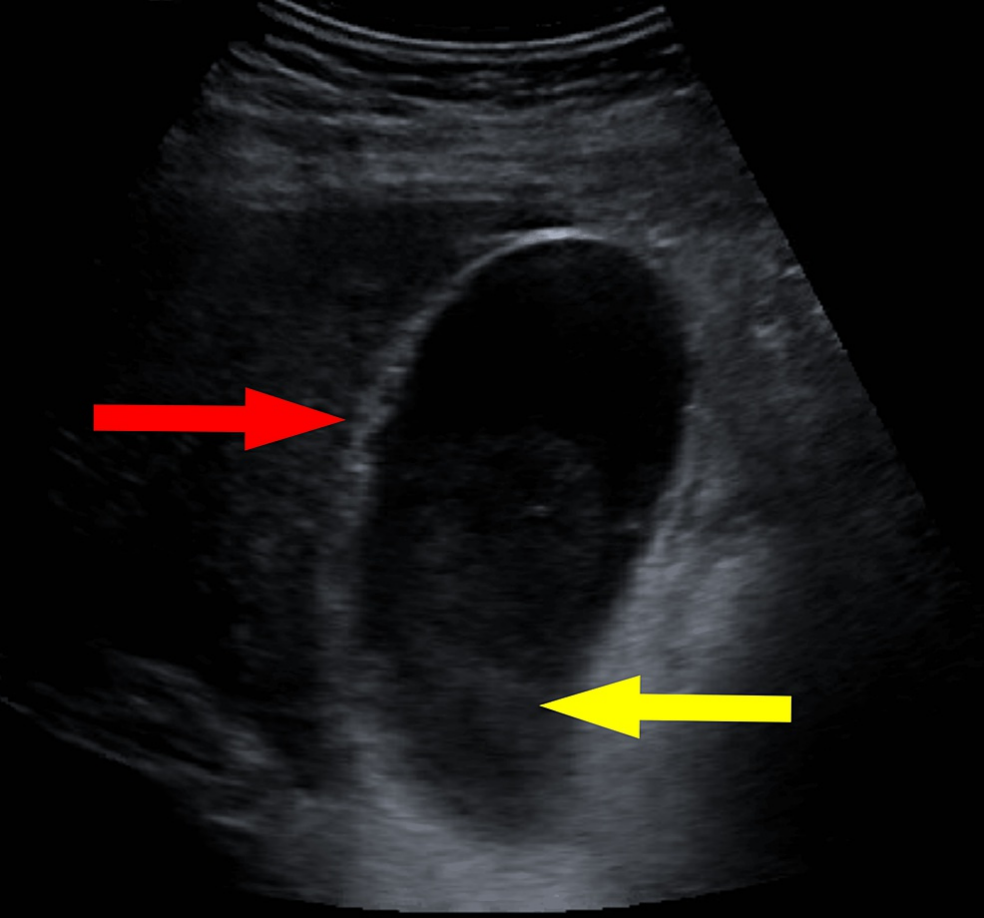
Ultrasound of the gallbladder Ultrasound of the gallbladder demonstrating sludge in the gallbladder (yellow arrow), mild wall thickening (red arrow), and absence of echogenic stone. These findings are suggestive of AAC
